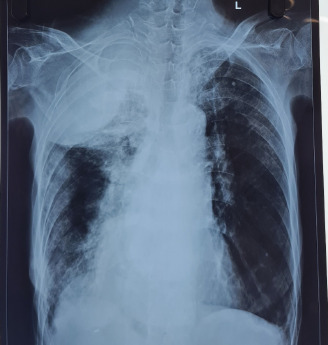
Chest radiographs of the same patient taken a year apart, both demonstrating the presence of a retained guidewire following central venous catheterization (Case 3)
